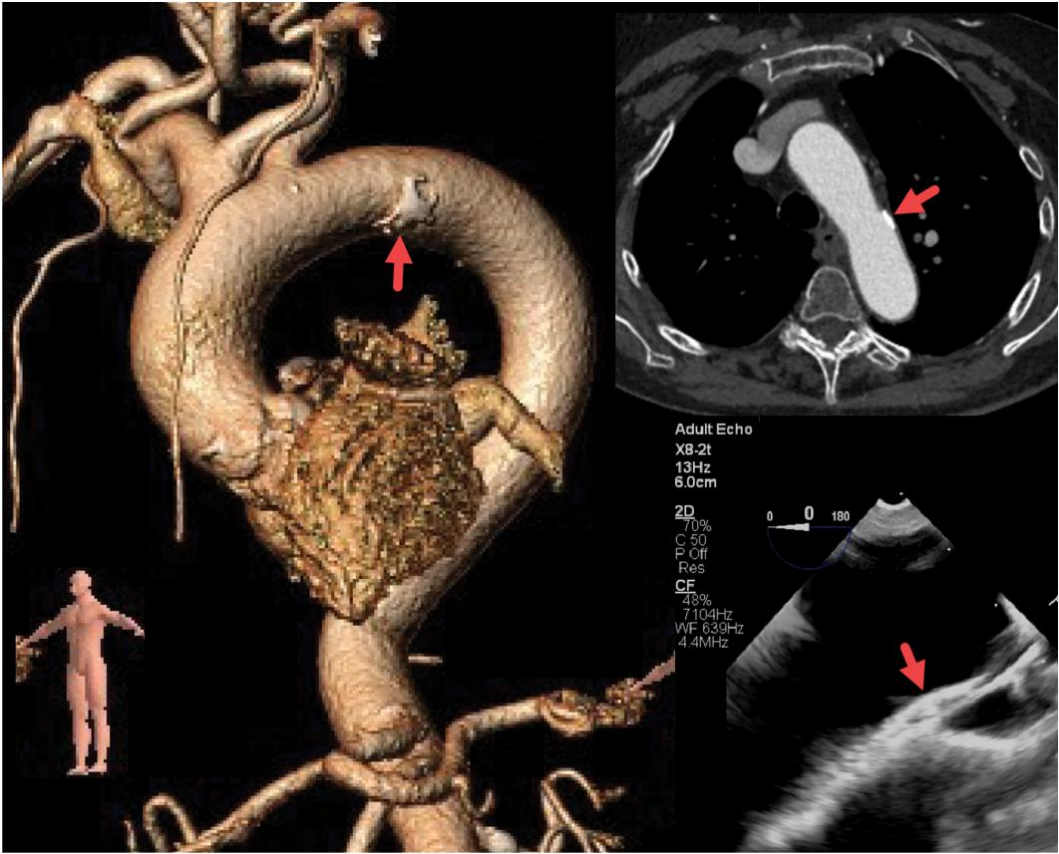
A focal atheroma was present in the distal arch on 3D rendering (left) and 2D (right upper) CT images. The aorta is unfolded. A <4 mm thick plaque was also visualized on a 2D long-axis TEE view of the arch (bottom right).
